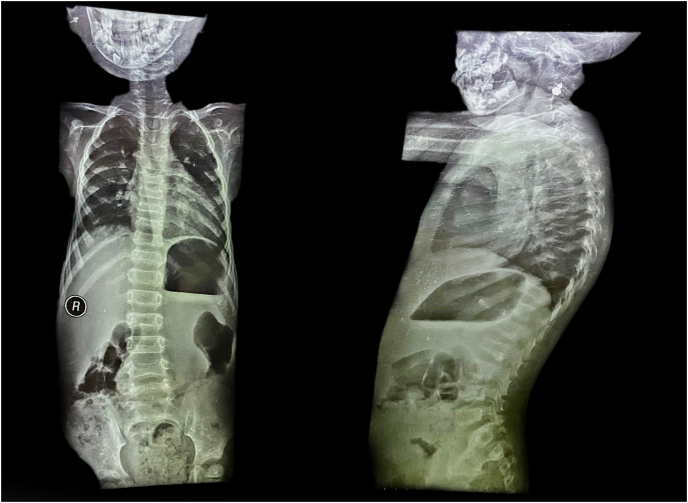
A spine X-ray in the lateral position and anterior-posterior position showing the scoliosis and kyphosis that the patient has.
